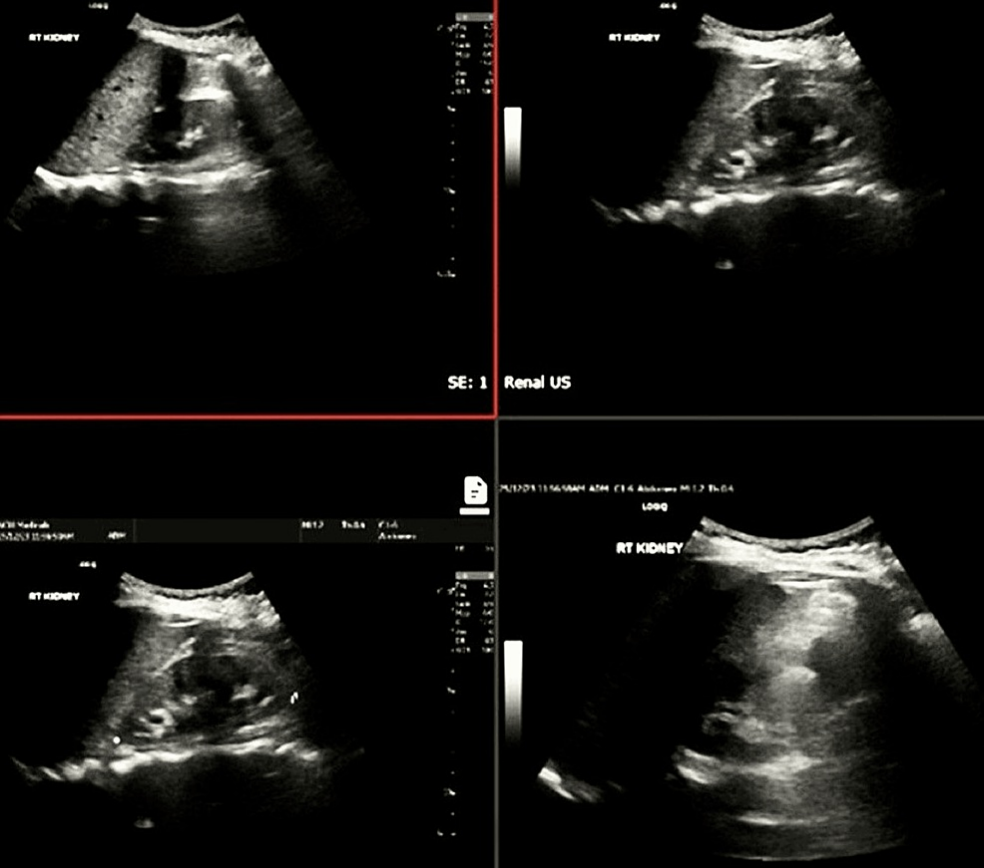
Renal ultrasound showing mild left renal hydronephrosis. Otherwise, unremarkable scan.
